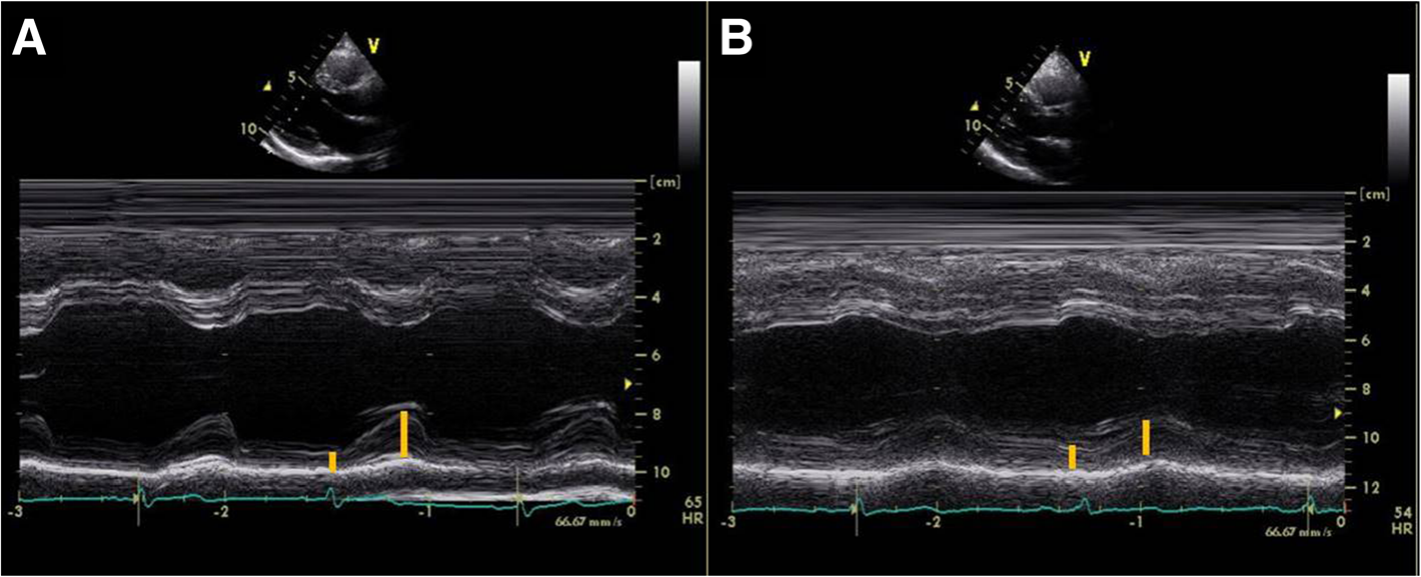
Echocardiographic images of M-mode from parasternal long-axis view, a patient with normal diastolic wall strain (DWS, a) and a patient with decreased DWS (b)
